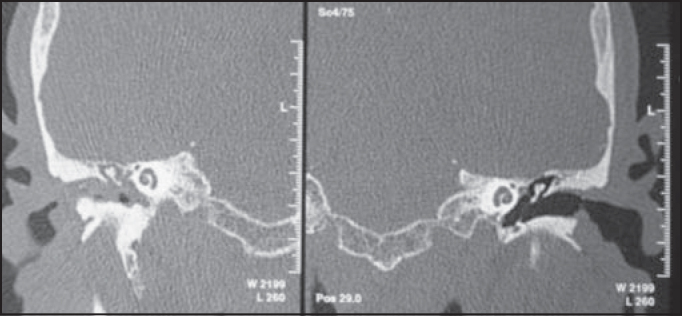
Temporal bone CT scan at coronal section evidencing complete obstruction of external auditory canal and right middle ear with soft part density and obliteration of oval and round window niches, preserved Chaussé spore. Left external and middle ears and both inner ears without any affections.
